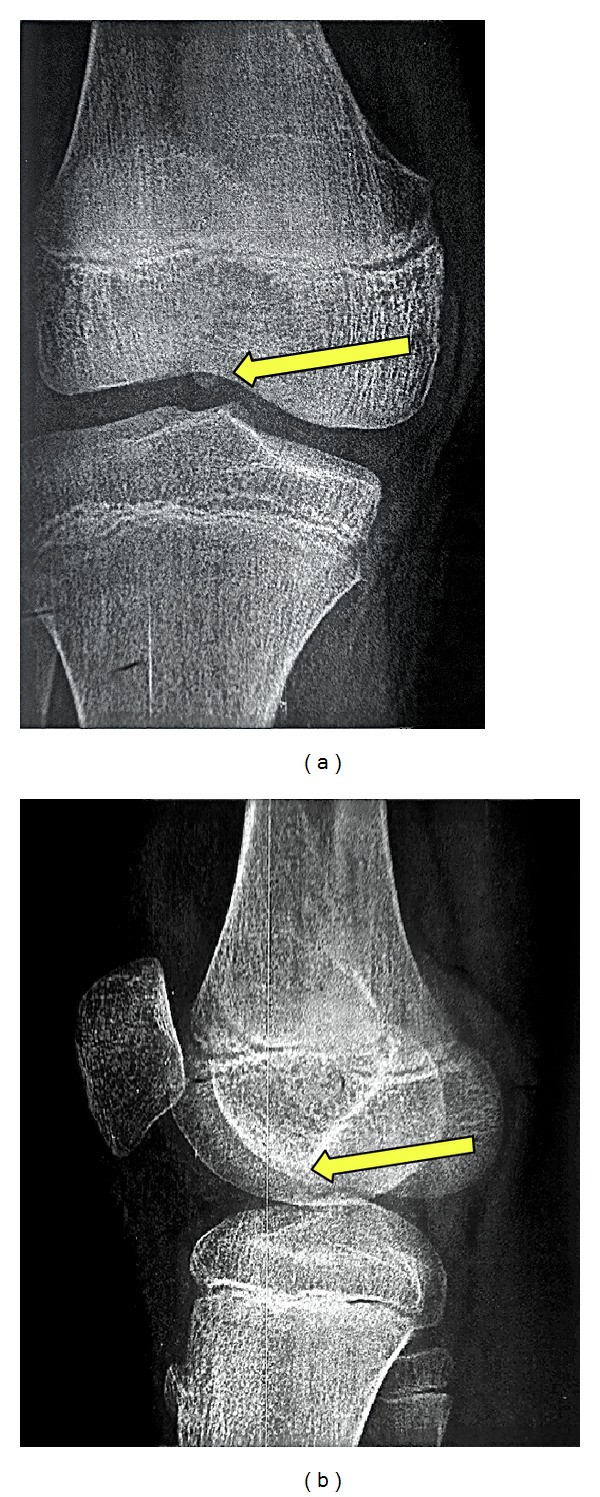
X-ray: (a) AP view and (b) lateral view showing what appeared to be a foreign body in the joint.
